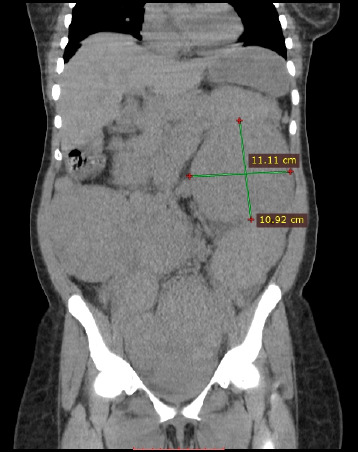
Coronal reformatted abdominal CT scan Images showing multiple isodense round and oval-shaped lesions in the peritoneal cavity after GNRH therapy
